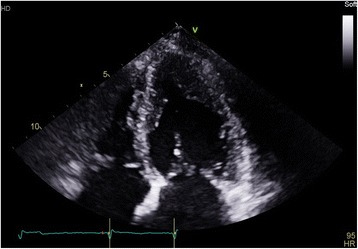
Apical four-chamber echocardiography showing apical left ventricular hypertrophy
